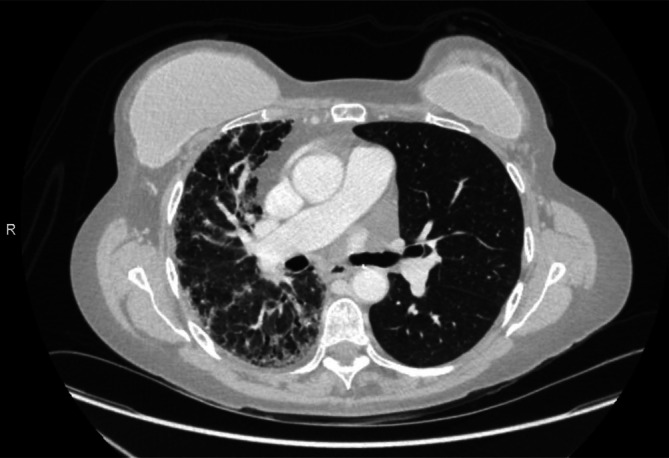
Axial contrast‐enhanced computed tomography scan of the chest demonstrating asymmetric lung findings. The right lung demonstrates diffuse interlobular septal thickening with areas of cylindrical bronchiectasis and moderate associated mosaic ground‐glass attenuation as well as honeycombing. Patient is status post left‐sided lung transplant.
